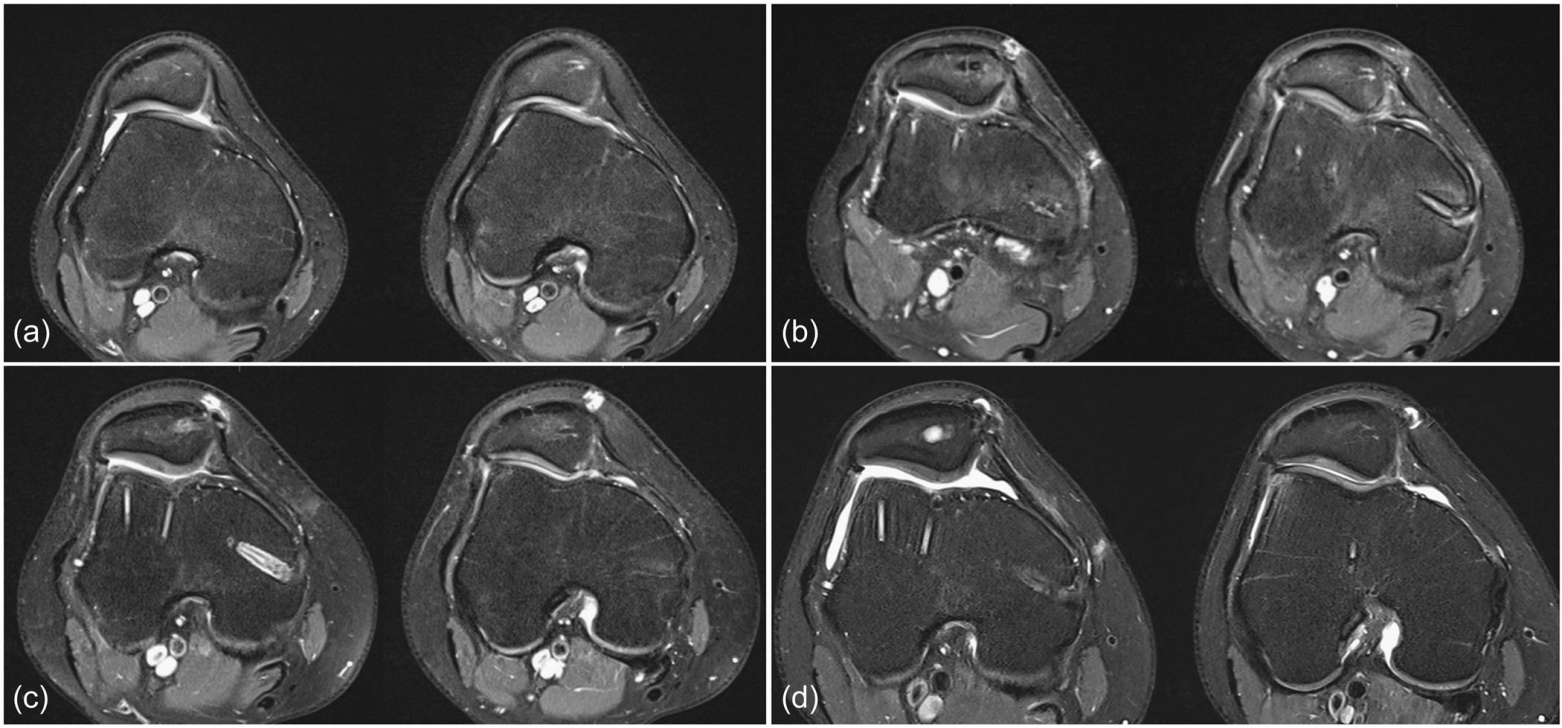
Patellofemoral cartilage evolution in a 14‐year‐old female patient. Initial magnetic resonance imaging (MRI) showed high‐grade dysplasia and signs of cartilage thinning at the lateral patellar facet (a). Eighteen months after trochleoplasty and MPFL‐R, patellofemoral congruence was improved (b). Follow‐up MRI at 35 months (c) and 112 months (d) showed an intact patellofemoral articular cartilage with no evidence of further degeneration. MPFL‐R, medial patellofemoral ligament reconstruction.
